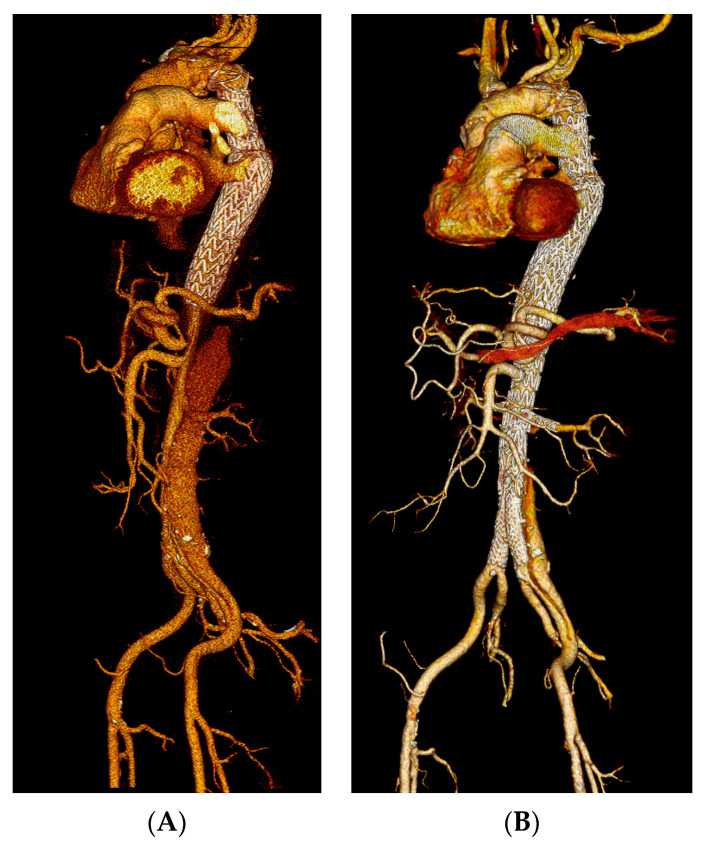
Postoperative CTA of a 67-year-old patient who received TEVAR after a FET procedure due to aortic diameter progression (A) followed by a distal completion with a fenestrated stent-graft 8 months later. (B) Note the entry tear in LEIA, which was left uncovered to reduce the risk of spinal cord ischemia.
